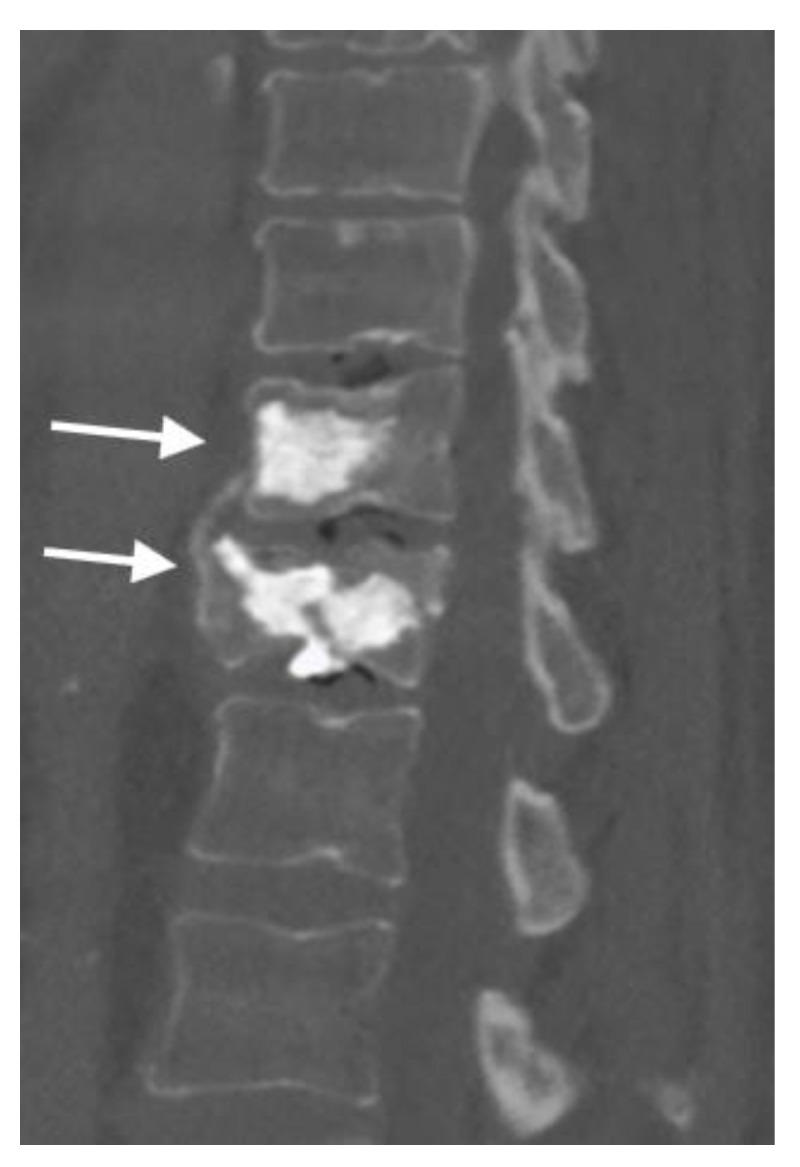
In the sagittal CT, the arrows show kyphoplasty for a T11 wedge compression fracture and acute traumatic comminuted T12 compression fracture, with mild height loss and no retropulsion.
